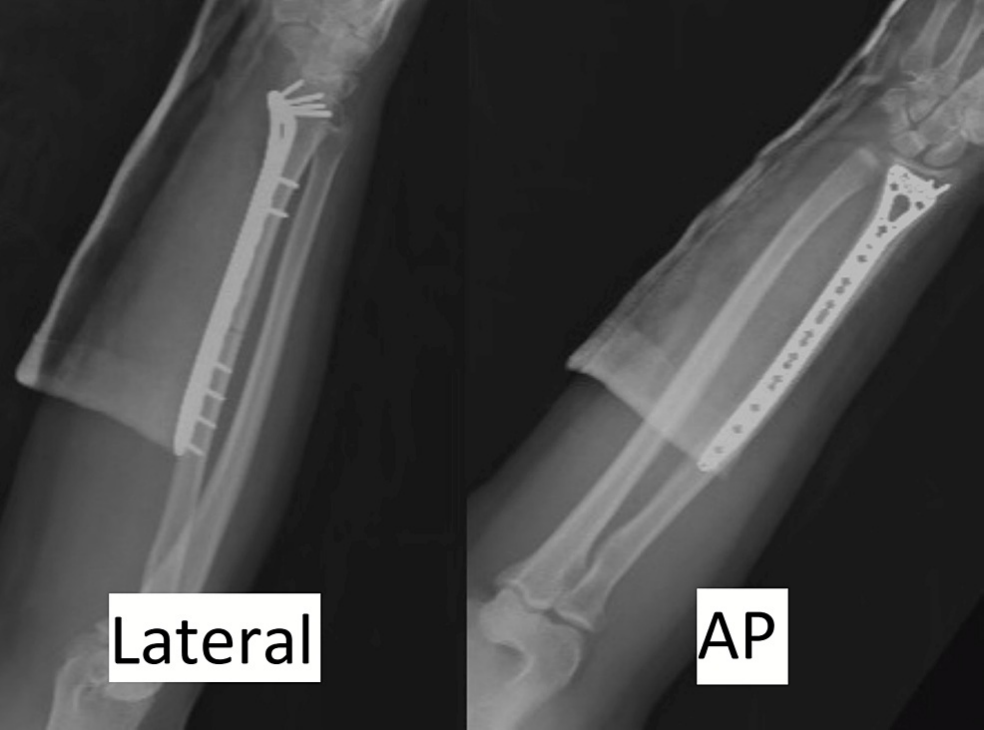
Postoperative anteroposterior (AP) and lateral view X-rays of the right forearm following open reduction internal fixation of a distal-third radial shaft fracture
